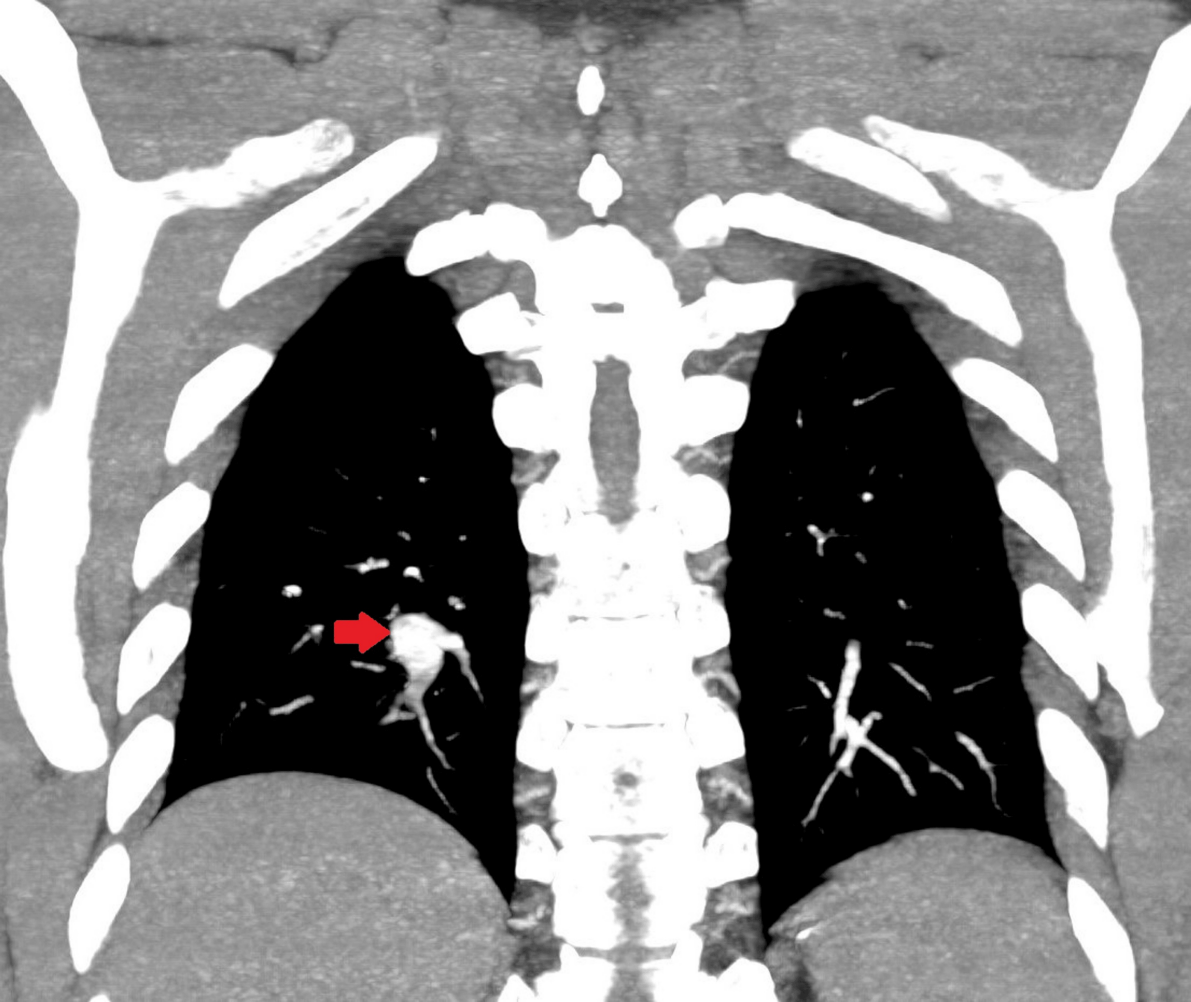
CT pulmonary angiogram (coronal view) demonstrating aneurysmal dilatation of the posterior segmental branch of the right lower lobe pulmonary artery
